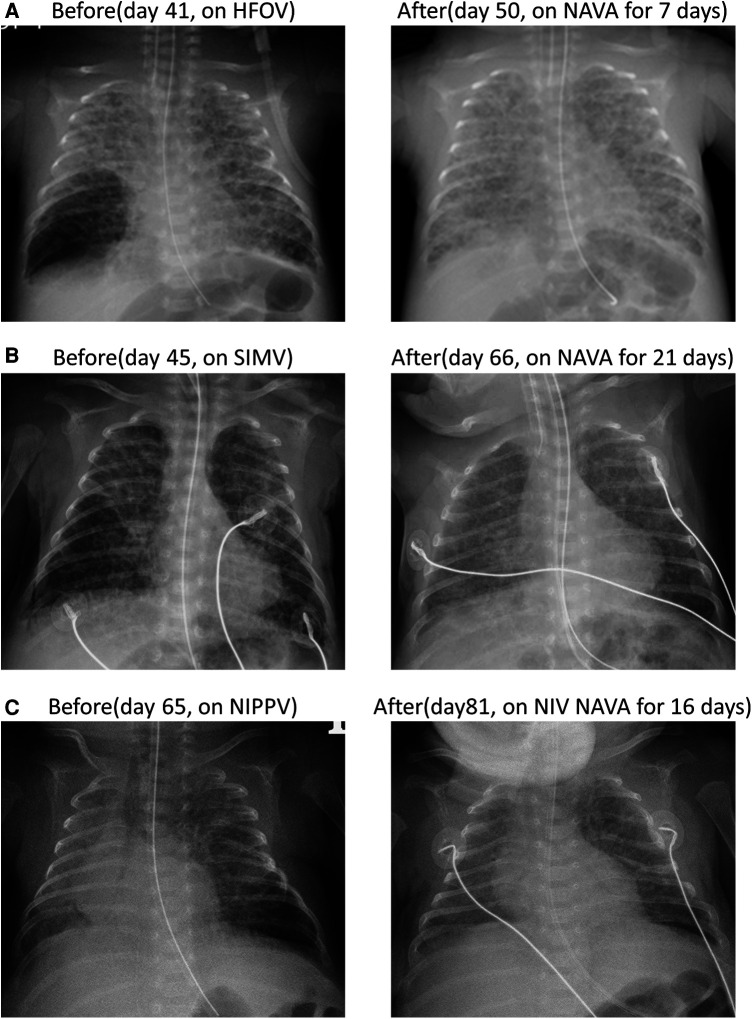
Three cases of localized PIE presented by anterior–posterior chest x-ray before and after NAVA. (A) In case 1, localized PIE over the right lower lobe was observed on day 43 and resolved on day 50 after 7 days of NAVA treatment. (B) In case 2, localized PIE over the right lower lobe presented on day 45 and resolved on day 66 after NAVA treatment for 21 days. (C) In case 5, localized PIE over left lower lobe on day 65 and completely resolved on day 81 after 16 days of NIV NAVA treatment.
